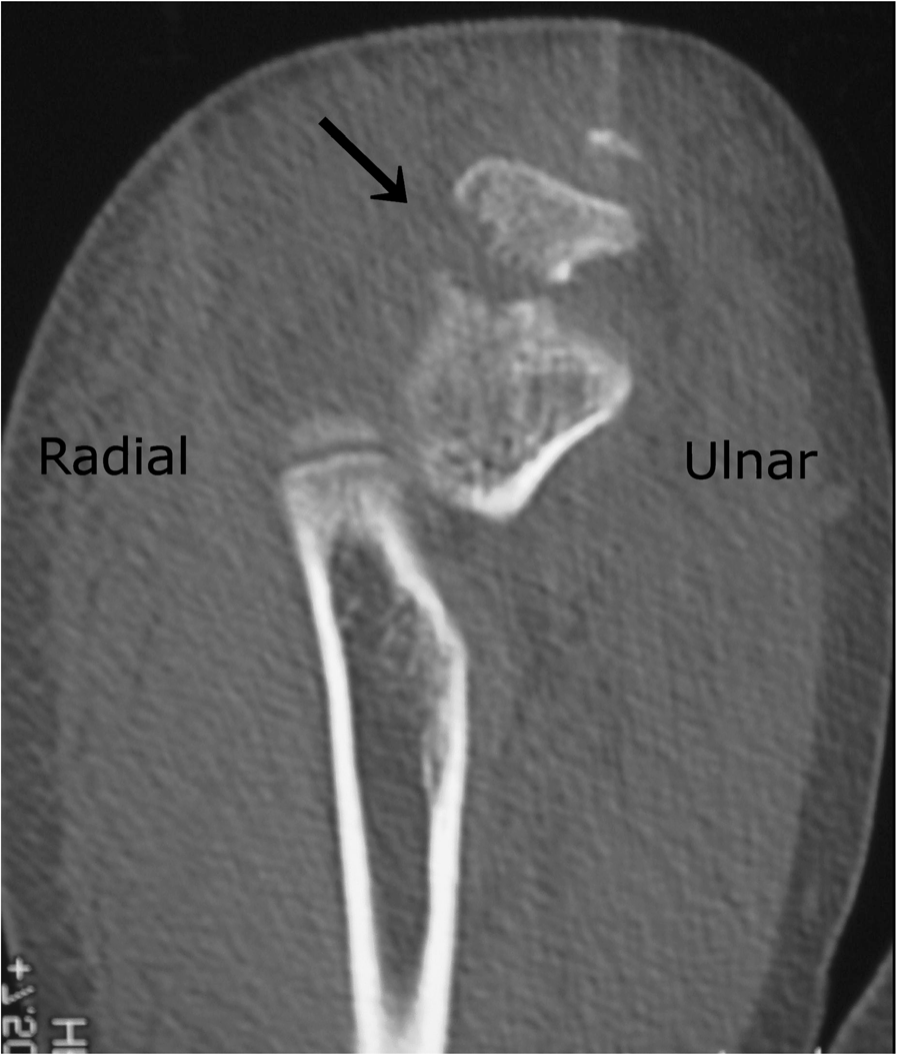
Computed tomography scan of the elbow showing the olecranon fracture line (arrow) running from proximal radius to distal ulnar.
